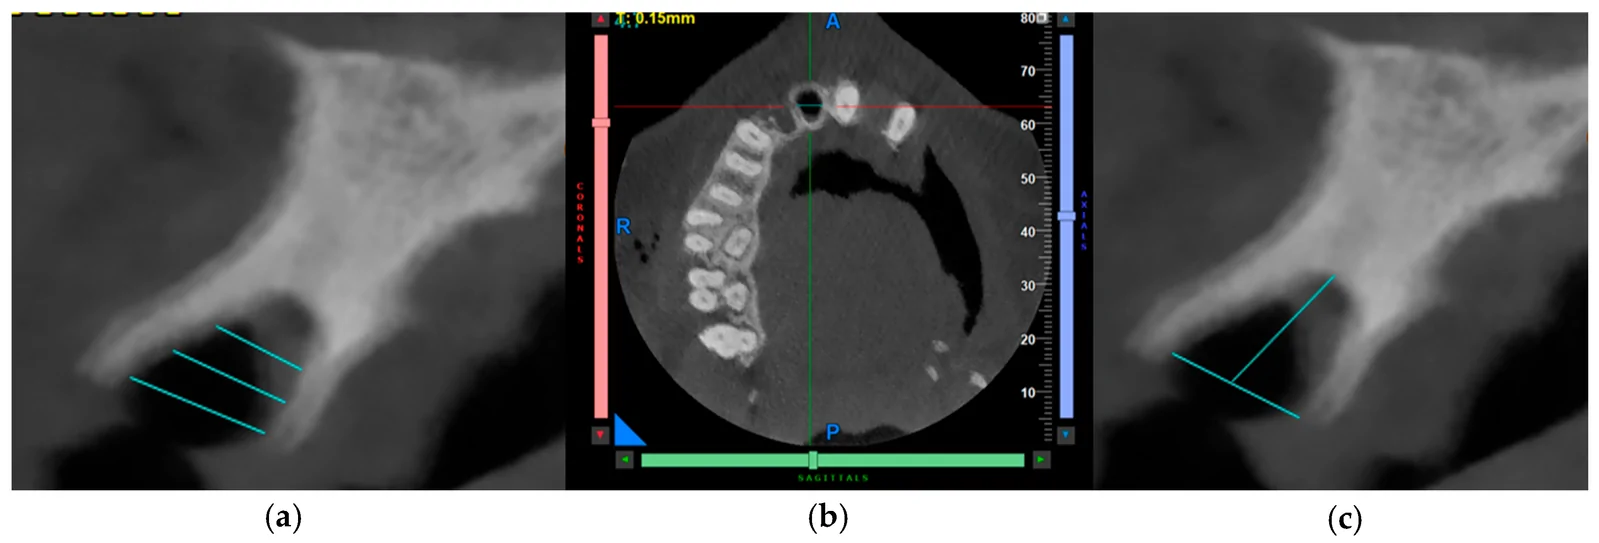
(a) Measurements taken of the empty socket using CBCT at the crest, 1 mm from the crest, and 3 mm from the crest; (b) mesiodistal dimension; (c) socket depth.
